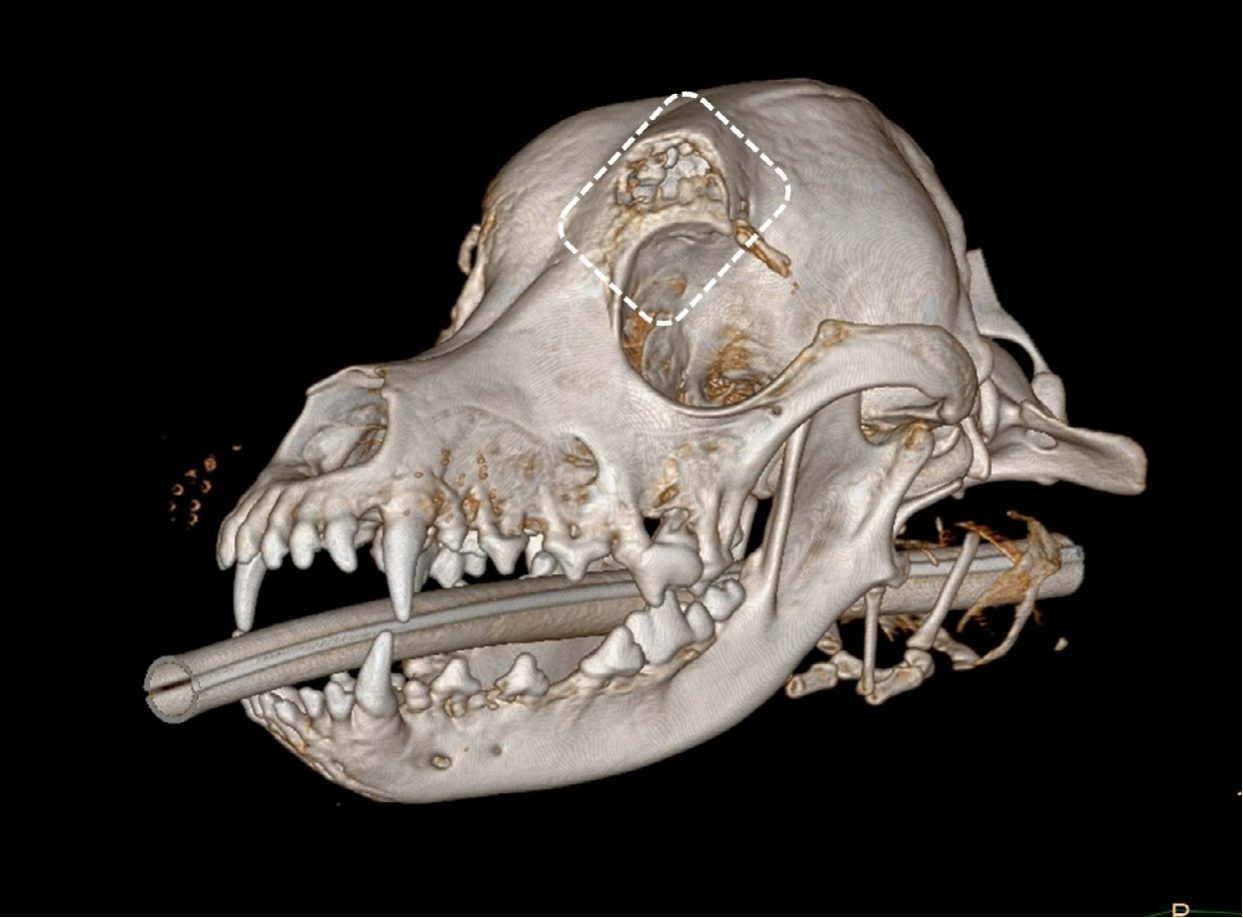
Computed tomography. 3‐D. Resected bones: part of the frontal bone, including the zygomatic process and medial part of the orbit.
